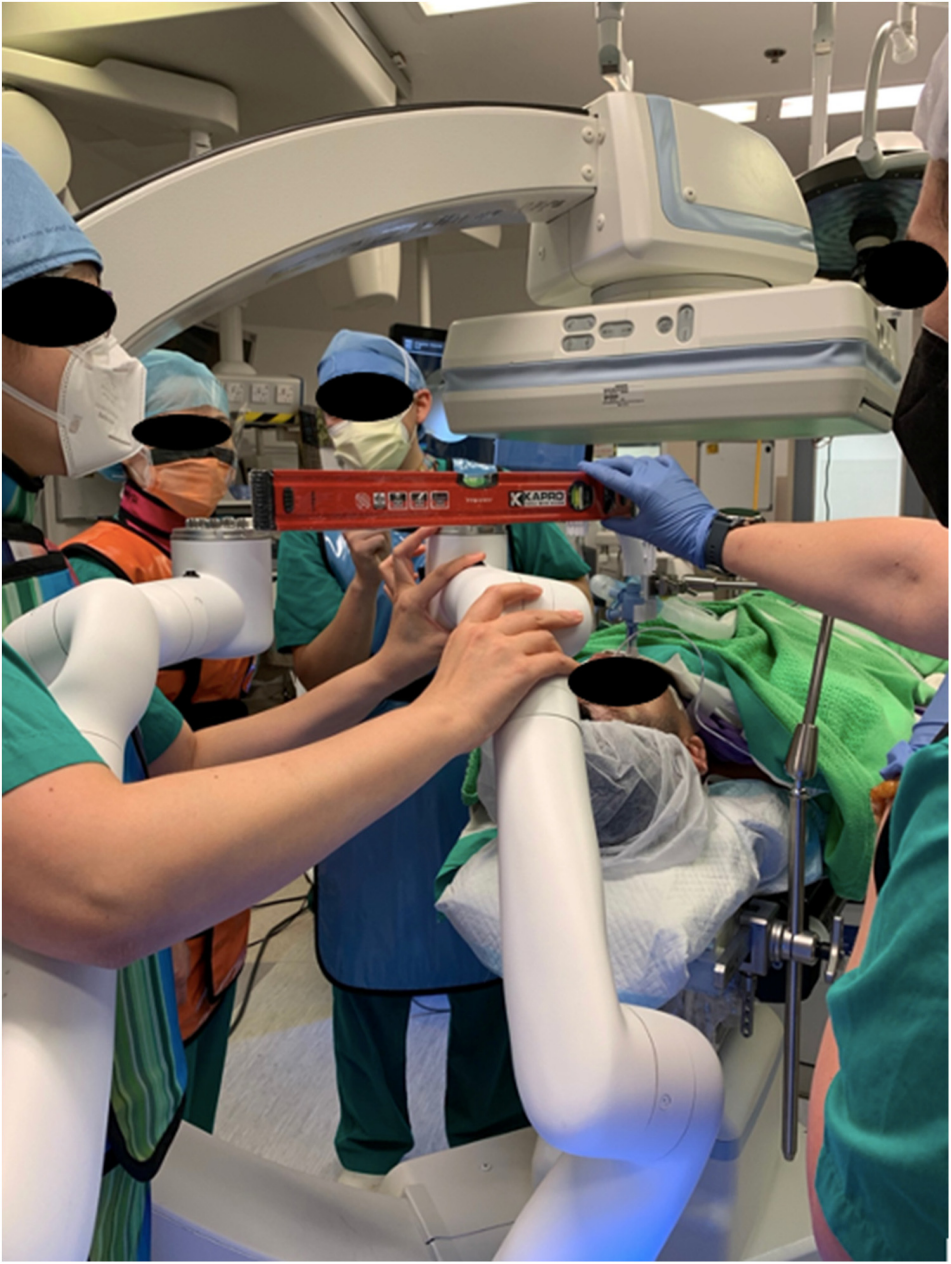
Setting up the monarch system to incorporate the Artis Zeego cone-beam CT (CBCT) as the adjunct imaging in the hybrid operating room (HOR). Careful alignment of the robotic arms is essentially for the accommodation of the CBCT.
